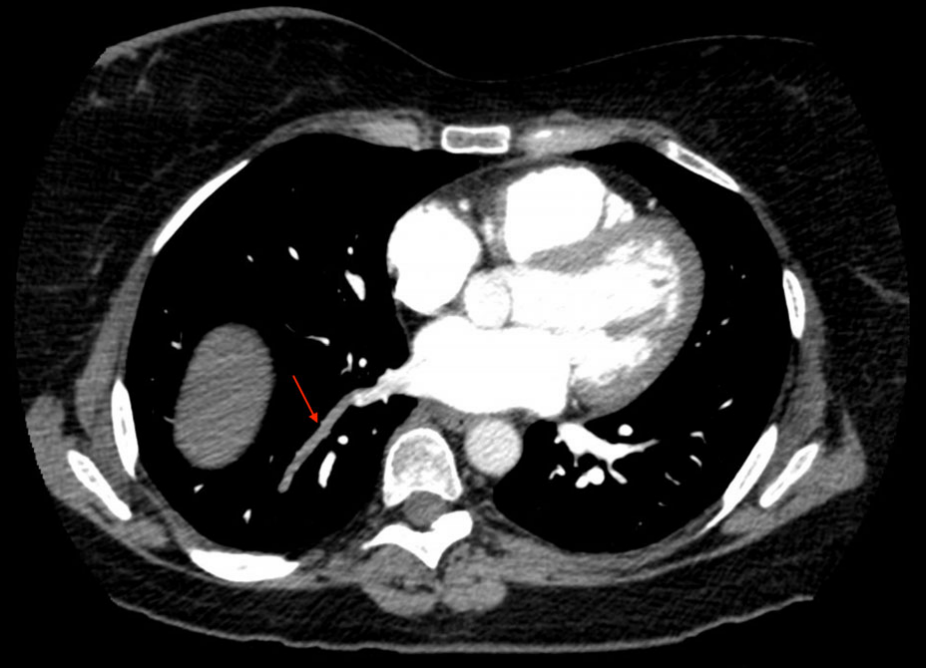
Axial view of a computed tomography (CT) pulmonary angiogram demonstrating a pulmonary vein thrombus (red arrow) involving the right lower lobe segmental vein.
